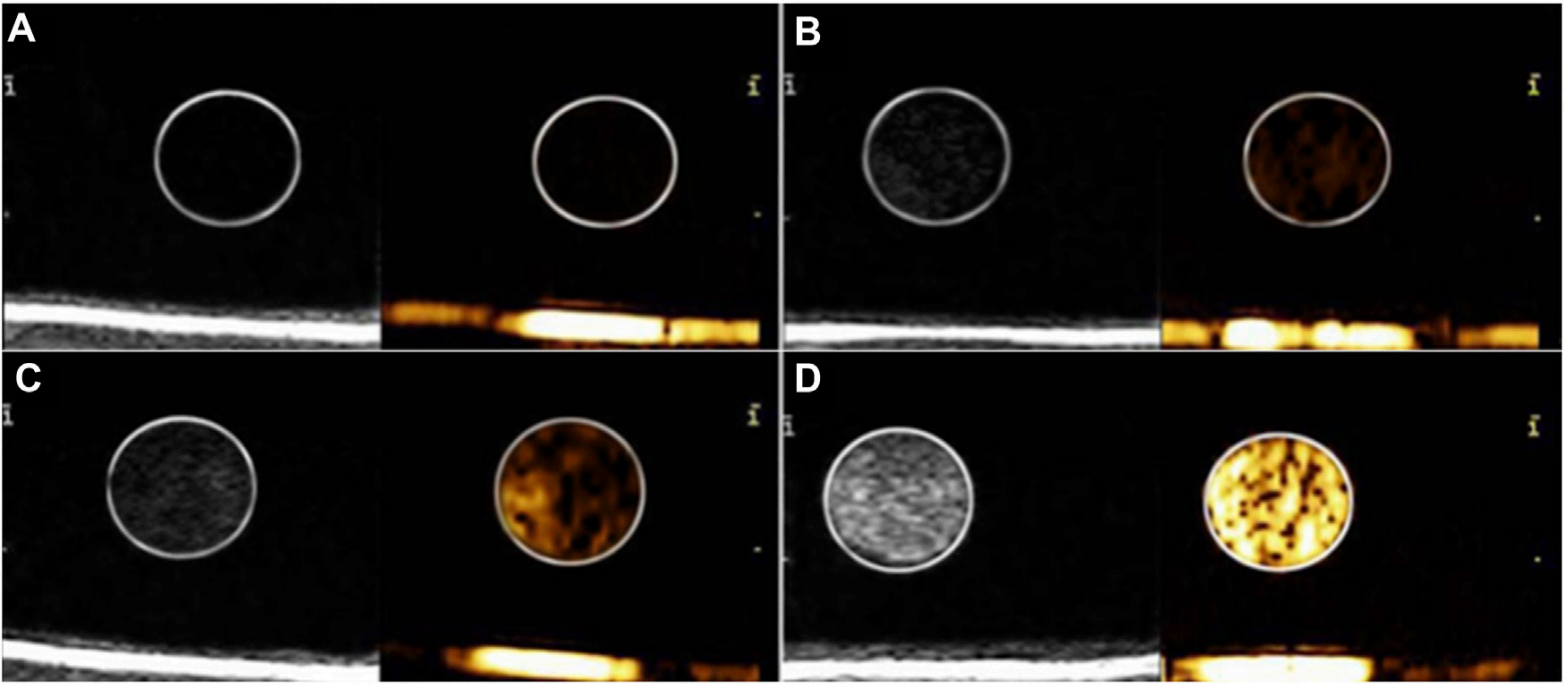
Ultrasound imaging of various concentrations of GSN-PLGA NPs. (A) Degassed water as the control group. (B) 0.25 mg/ml GSN-PLGA NPs. (C) 0.5 mg/ml GSN-PLGA NPs. (D) 1 mg/ml GSN-PLGA NPs.
